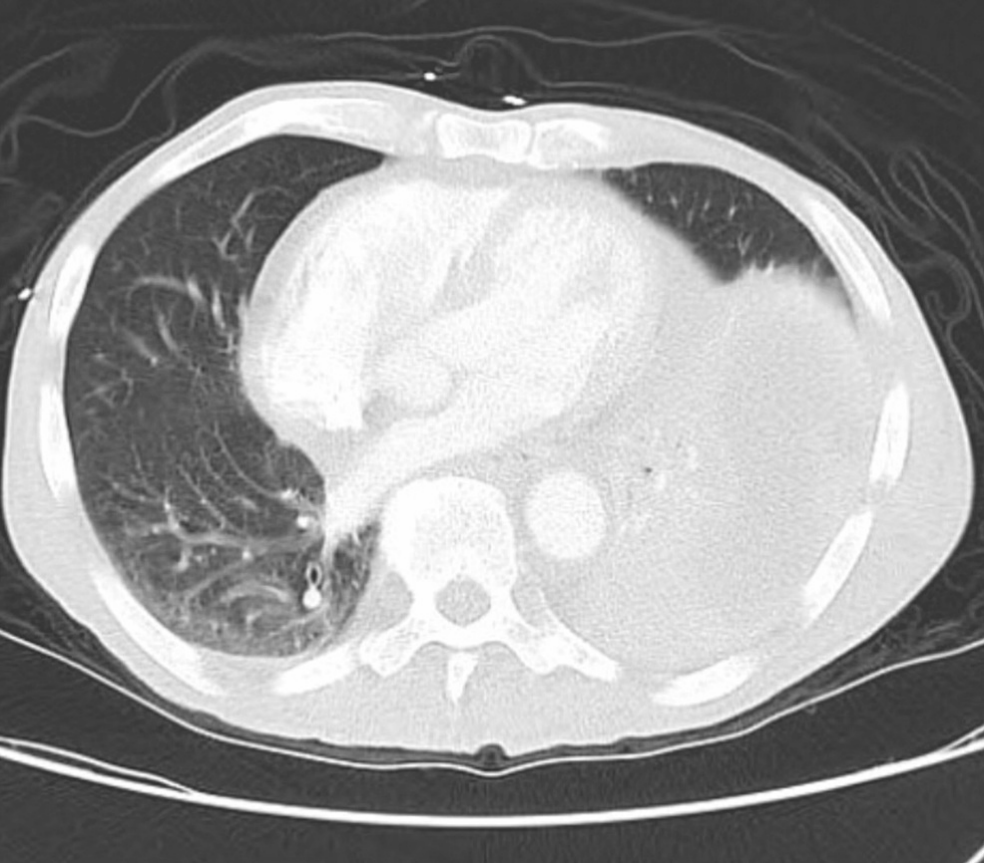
CT scan showing large pleural effusion and large abnormality, which was concerning for a pulmonary embolism arising from the pulmonic valve
